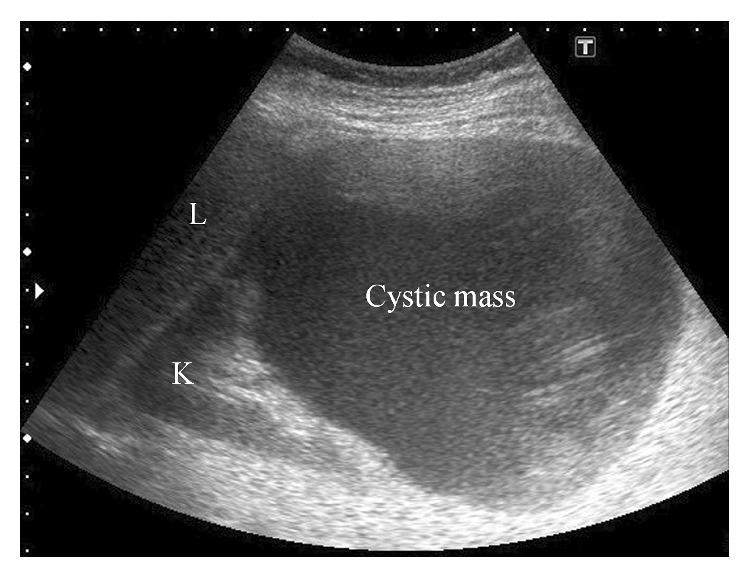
Ultrasonographic image of the right kidney, demonstrating a 12 × 10 cm cystic mass in the lower pole of the right kidney. L, liver; K, right kidney.
